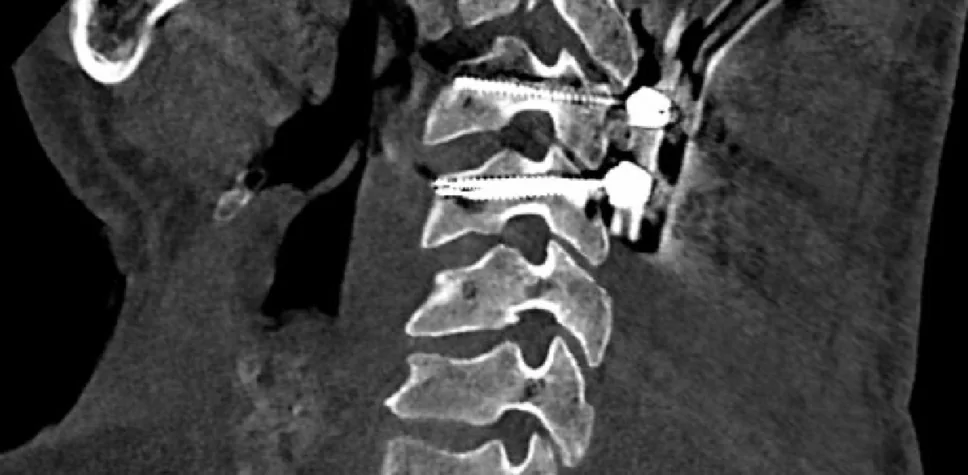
Sagittal CT pedicle screw C4-lt. (same patient as in figures 3–8). *C*,* cervical spine*
